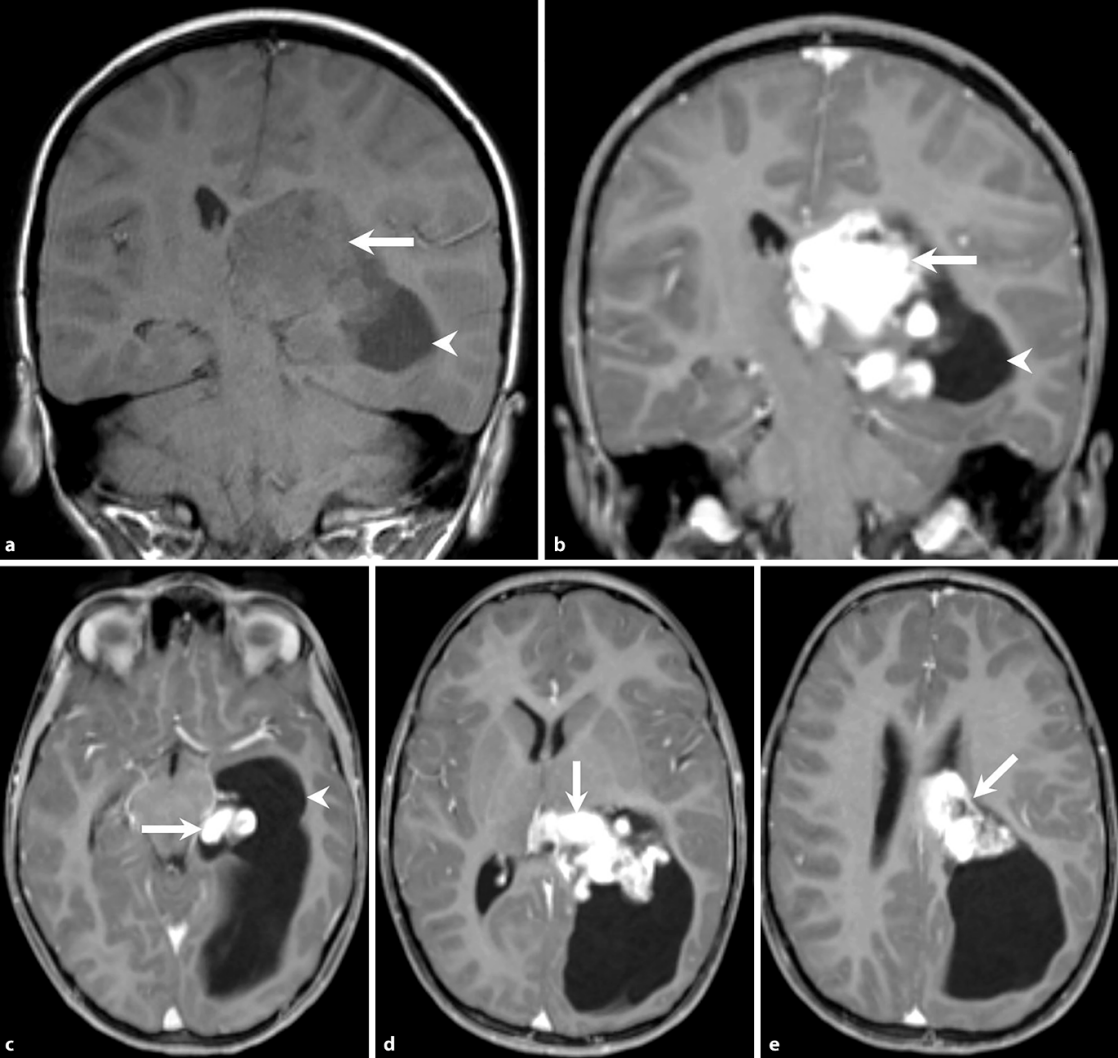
On coronal T1-weighted non-contrast images (a) the lesion has a relatively homogeneous appearance and seems isointense compared to the grey matter (a*arrow*). On coronal (b) and axial (c–e) T1-weighted images obtained after i.v. administration of gadolinium the lesion reveals marked and homogeneous contrast enhancement (*arrow*). The dilatation of the left lateral ventricle is again clearly visualized (a–c*arrowhead*)
